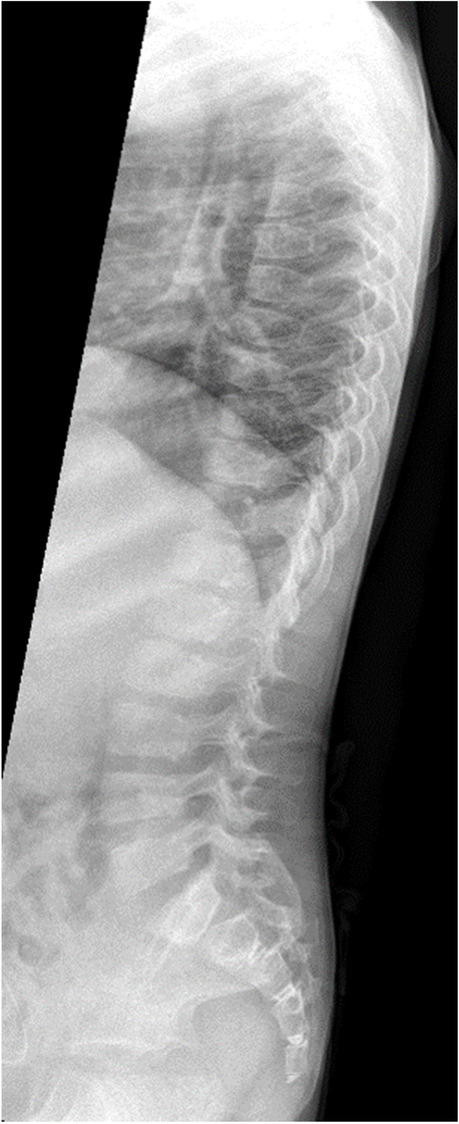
Lateral spine radiograph for case 2 showing prominent thoracic kyphosis and generalized vertebral flattening
